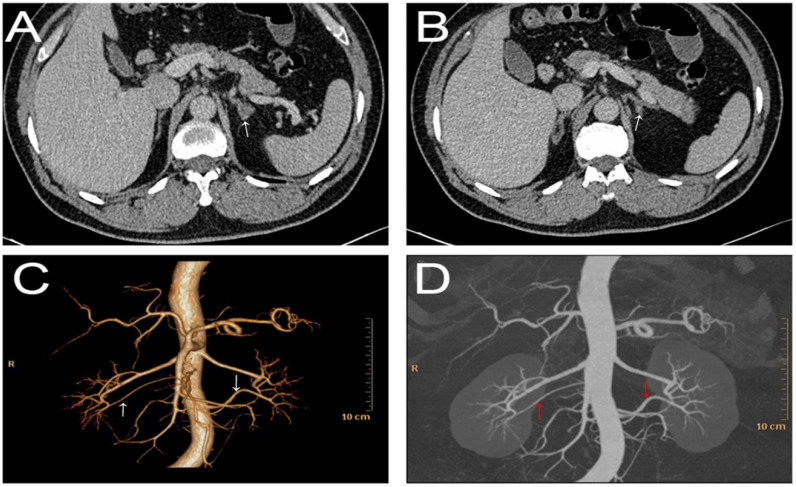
Adrenal contrast-enhanced CT. (A) The white arrow showed a round soft-tissue density mass with a diameter of 8 mm; (B) The white arrow showed the left inner branch adrenal gland was thickened. CTA showed no stenosis of the bilateral renal arteries and the presence of bilateral renal accessory arteries (pointed by the white arrow in (C) and red arrow in (D)).
